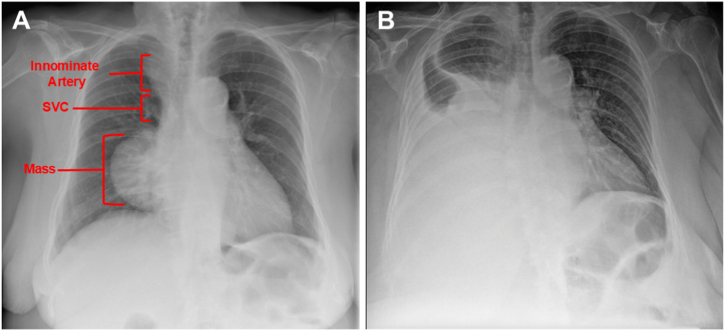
Radiographic findings of ruptured thymoma. Chest roentgenogram, posterior-anterior (PA) view obtained one week prior to admission (A) and chest roentgenogram, anterior-posterior (AP) view on the day of surgery (B), showing a new right pleural effusion with mild mediastinal shift to the right.
